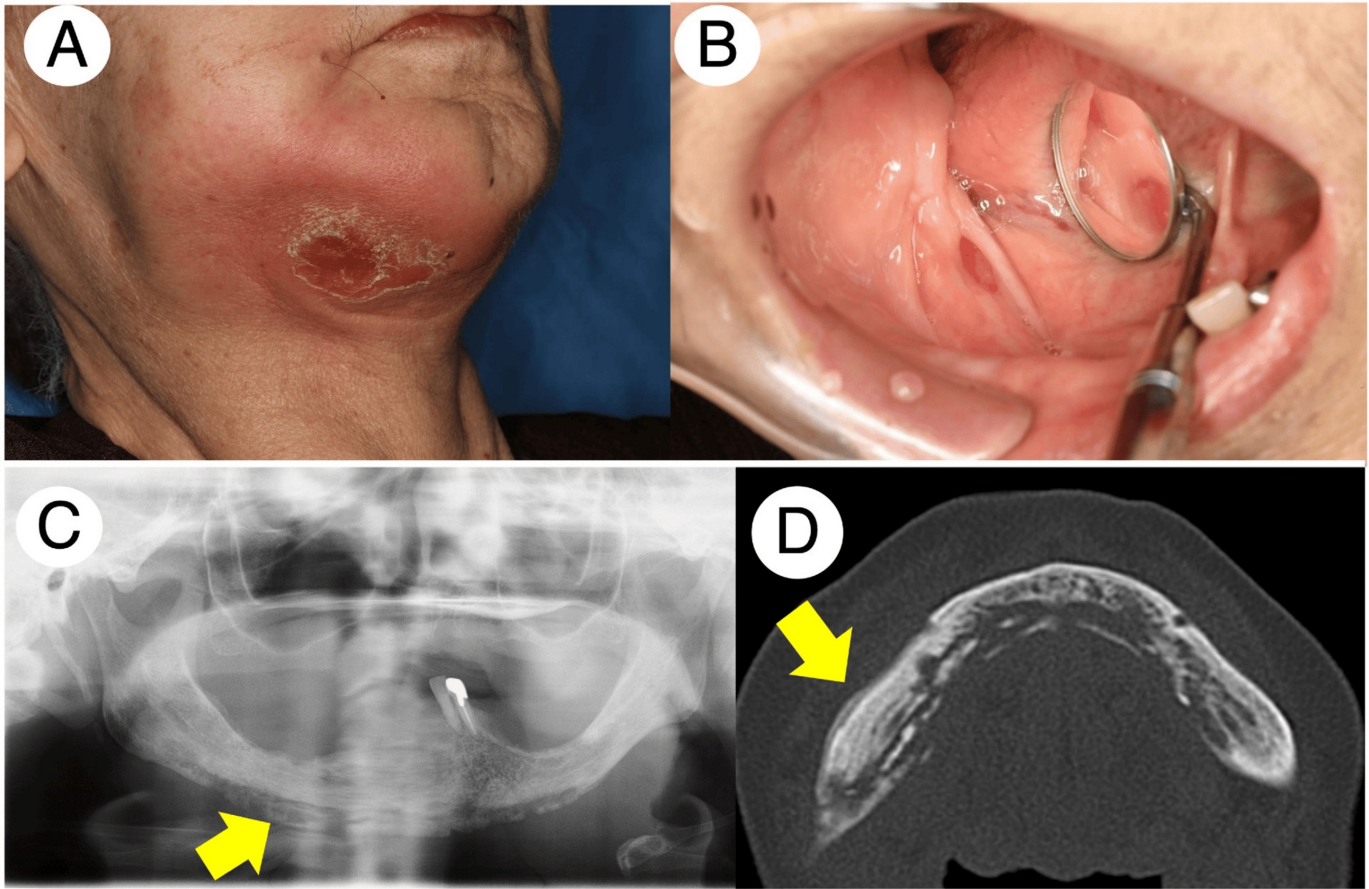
Patient 3 clinical and radiographic findings before the treatment. Extraoral examination found a significant swelling of the mandibular skin with redness (A). Intraoral examination identified abscess formation in the right-sided alveolar ridge with exposed bone probing through the fistula (B). Panoramic X-ray and MDCT exhibited evident signs of periosteal reaction throughout the mandibular edge (the yellow arrow indicates the finding of periosteal reaction) (C and D). MDCT: multidetector computed tomography
